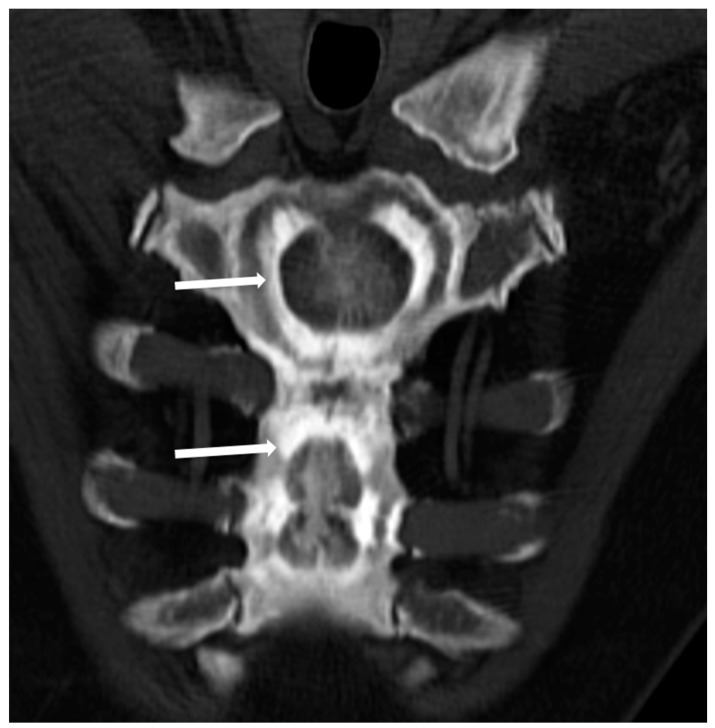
Computed Tomography (CT, coronal-oblique reconstruction) of the sternum in a 48-year-old male affected by osteopetrosis (ADO type 2). The so-called ‘bone in bone’ appearance is detectable with a well-defined increased density within the skeletal segment involved (arrows).
